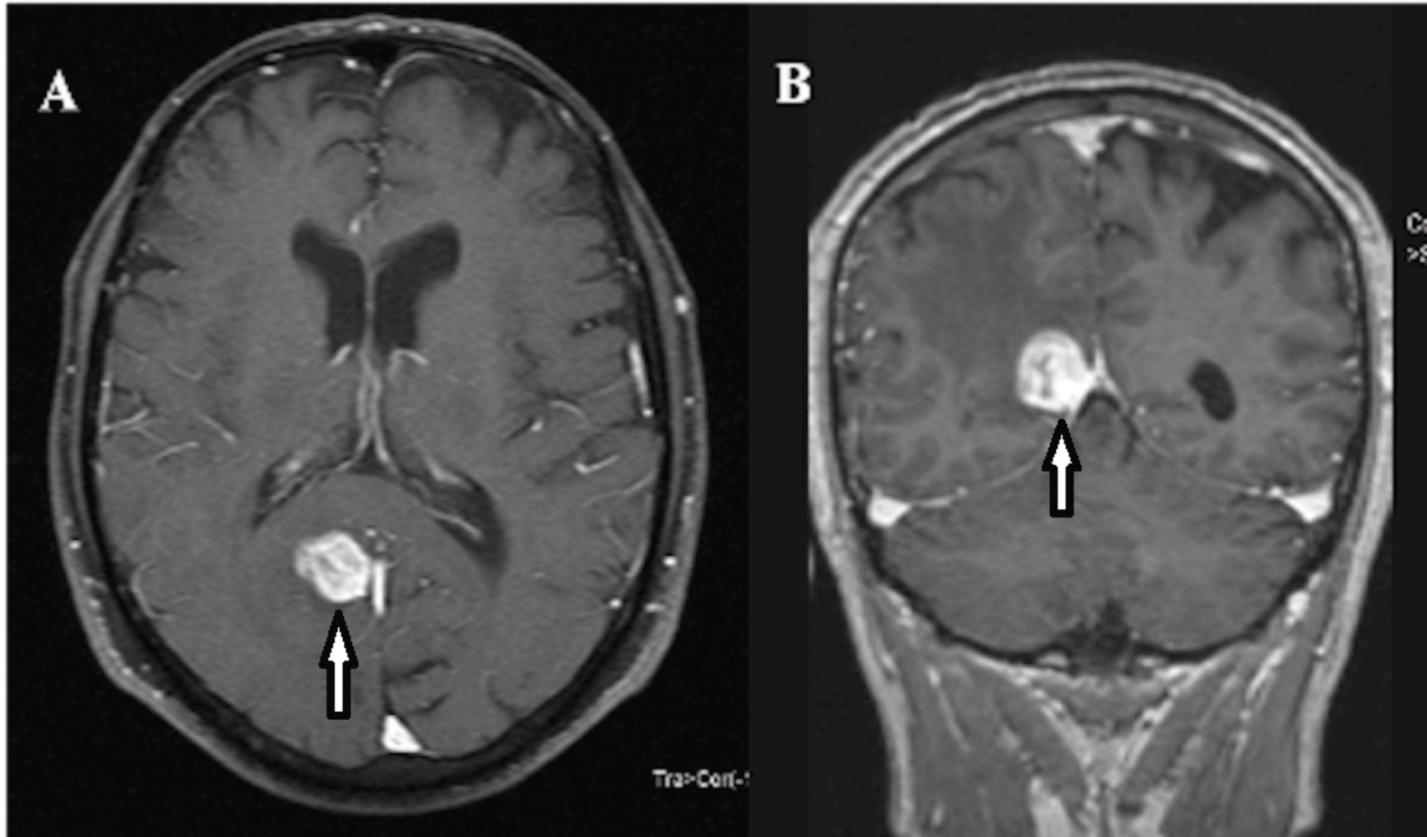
Axial (A) and coronal (B) post-contrast T1-weighted cranial MRI sections, revealing a 15x15 mm enhancing lesion in the right occipital region (white arrows), which corresponds to the previously identified iodine-positive focus.
